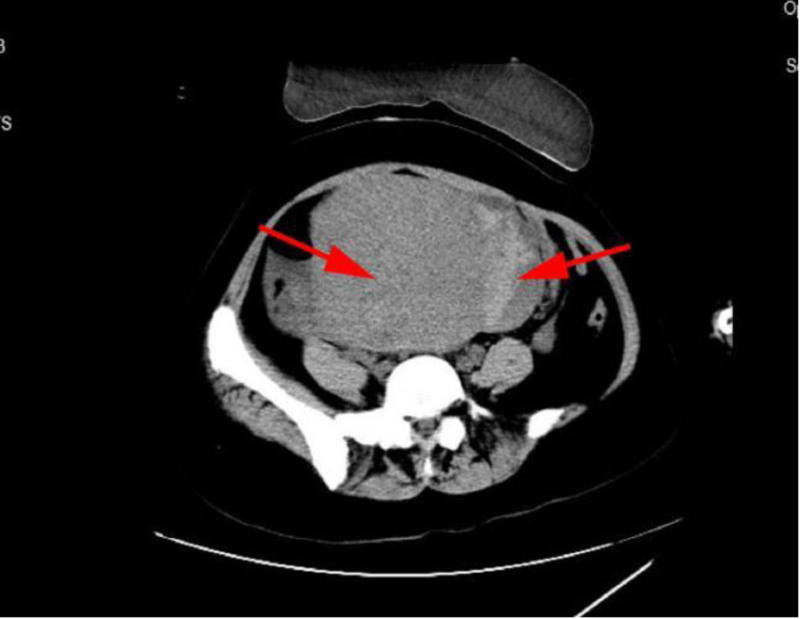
The CT scan revealed adenomyosis in the lower part of the uterus (left arrow), along with hematoma adjacent to it (right arrow). CT = computerized tomography.
